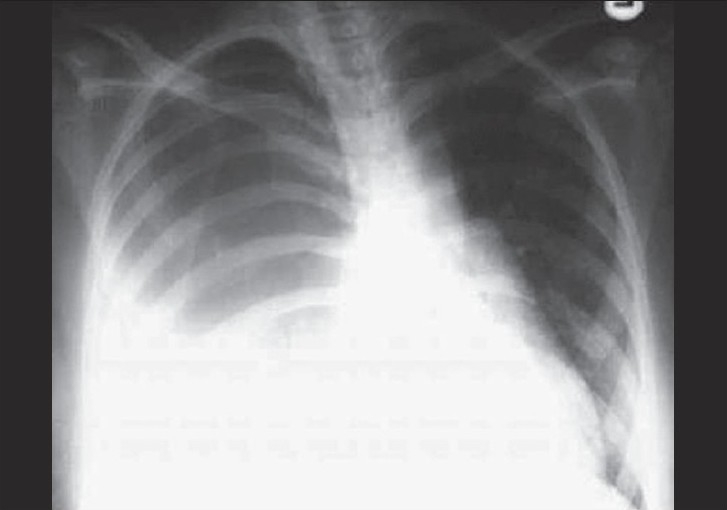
Chest X-ray shows homogenous opacity present at the middle and lower zones of the right lung obliterating the outline of the right dome of the diaphragm and the right CP angle suggesting pulmonary consolidation with large right pleural effusion. Left lung is clear
